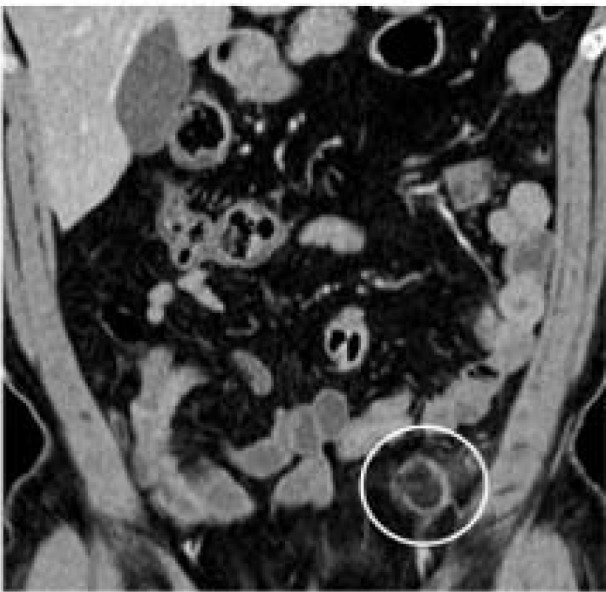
Longitudinal abdominal CT with contrast enhancement demonstrating primary epiploic appendagitis adjacent to the sigmoid colon 7 months prior to presentation
